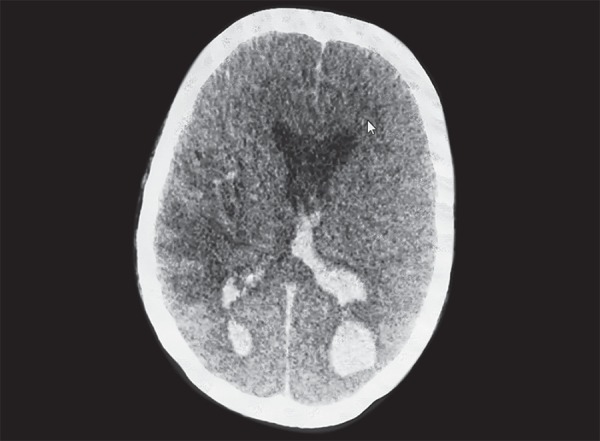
CT brain showing intracerebral bleed with ventricular extention
